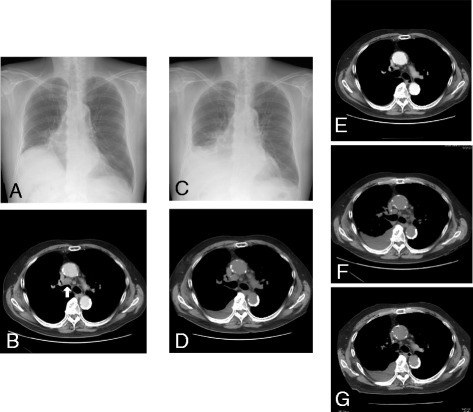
Chest radiograph and chest CT scan before (A and B) and after (C, D, E, F and G) crizotinib treatment: (A) Chest radiograph before crizotinib therapy, (B) and swelling of the mediastinal lymph node (#4R), (C) chest radiograph after 5 days of crizotinib treatment revealing increase in pleural effusions, (D) detection of right pleural effusions due to the appearance of congestive heart failure and renal failure, (E) disappearance of pleural effusions and significant shrinking of the mediastinal lymph node. (F) CT scan just before the crizotinib re-challenge after 4 months withdrawal. (G) CT scan after 3 months of the crizotinib re-challenging treatment.
